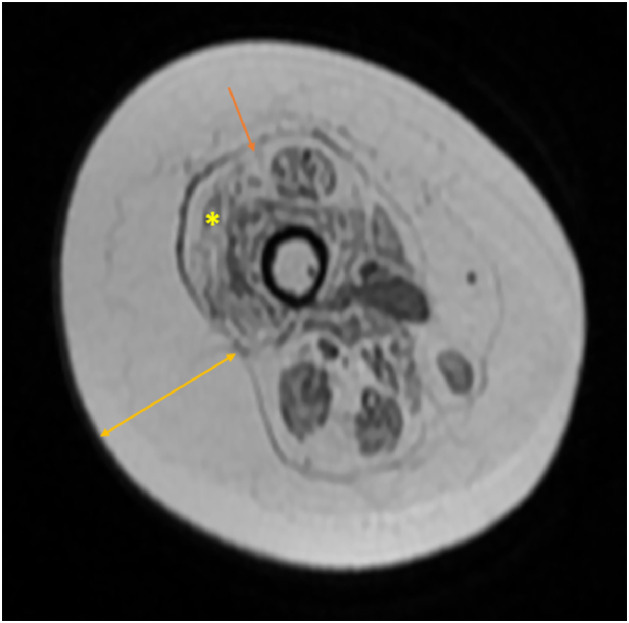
Axial T1-weighted MRI of the mid-thigh demonstrating disease-specific muscular alterations in SMA, including marked atrophy and fatty degeneration of the vastus lateralis muscle (*), blurring of the intermuscular septum (arrow), and increased subcutaneous fat thickness relative to reduced muscle bulk (double-headed arrow).
